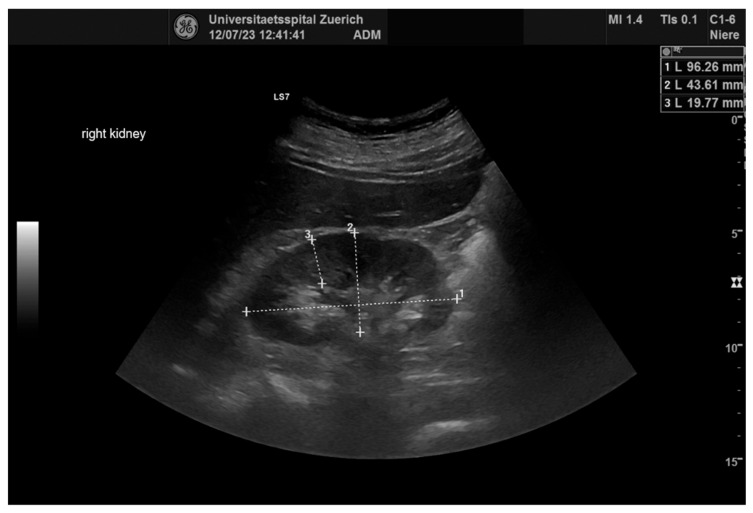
B-mode renal ultrasound right kidney. 1: length of kidney, 2: width of kidney, 3: width of kidney parenchyma.
